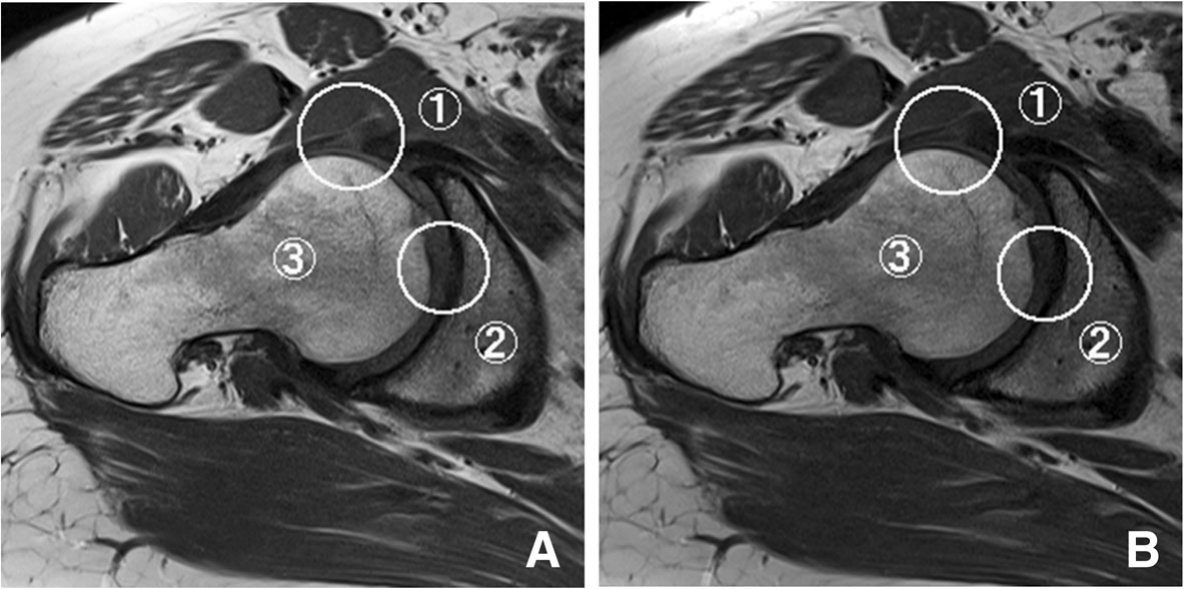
A 50-year-old woman with right hip pain. Oblique axial T1-weighted images with conventional (a) and simultaneous multi-slice sequences (b). Circles indicate areas for image evaluations: ➀ acetabular labrum, ➁ articular cartilage, ➂ bony trabecula
